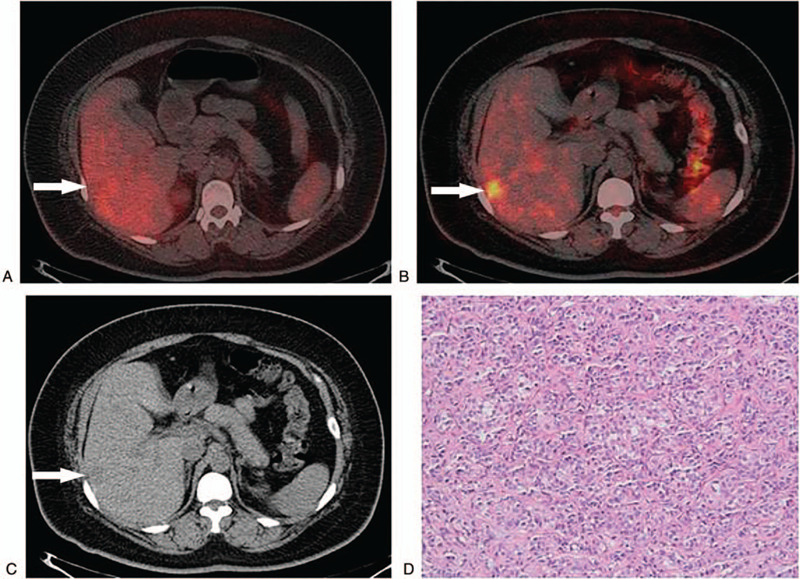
(A). Conventional positron emission tomography/computed tomography fusion imaging showed that the fluorodeoxyglucose uptake level of the lesion in the right lobe of the liver (indicated by the white arrow) was similar to that of normal liver tissue, standard uptake maximum value was 4.5. (B) Two-hour delayed positron emission tomography/computed tomography fusion imaging showed that the fluorodeoxyglucose uptake level of the lesion in the right lobe of the liver (indicated by the white arrow) was significantly higher than that in normal liver tissue, standard uptake maximum value was 7.5. (C) Plain computed tomography imaging showed that the lesion in the right lobe of the liver (indicated by the white arrow) was a quasi-circular and low-density lesion with unclear boundary, the diameter was about 2.6 cm. (D) Final pathological results suggested metastatic adenocarcinoma.
